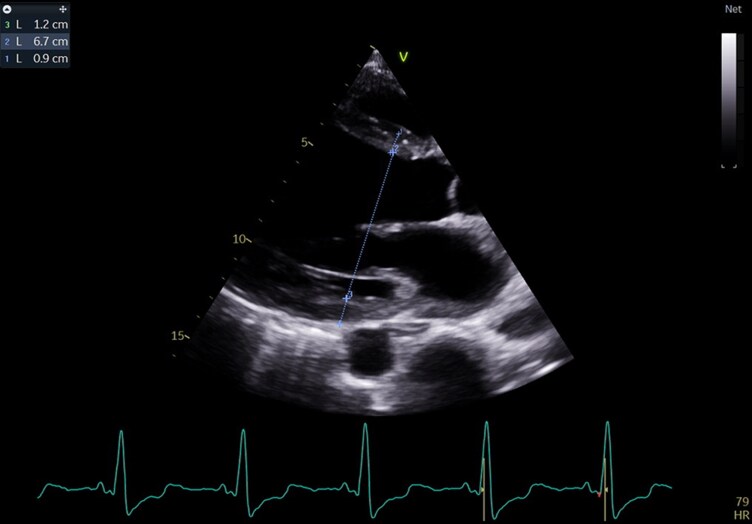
Parasternal long axis view in end-diastole. Dilated left ventricle (left ventricular end-diastolic diameter = 67 mm; 42 mm/m^2^).
